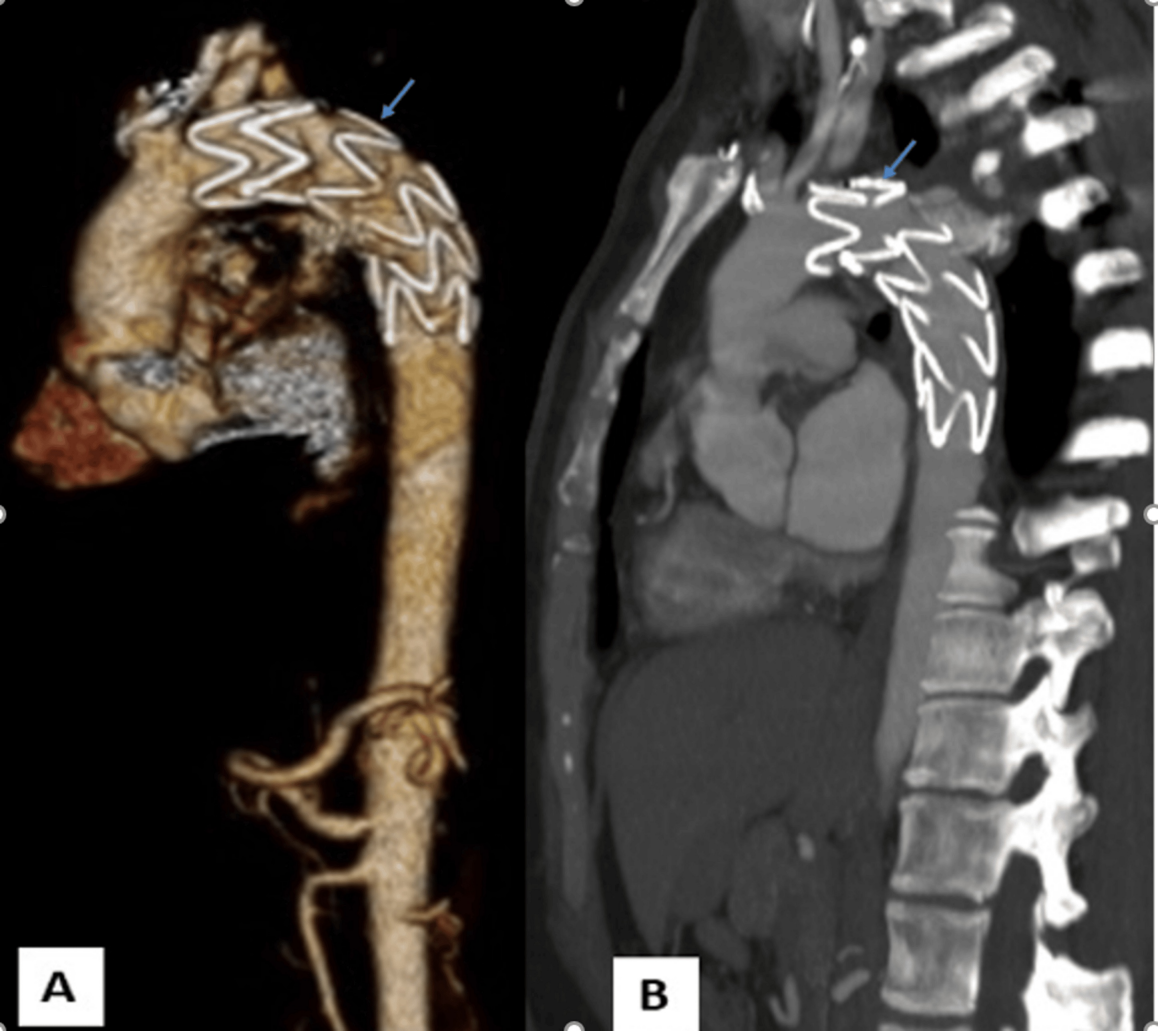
Post-treatment CT-angiography 3D reconstructions views (A) and sagittal (B): aortic stent-graft in place (blue arrow)
